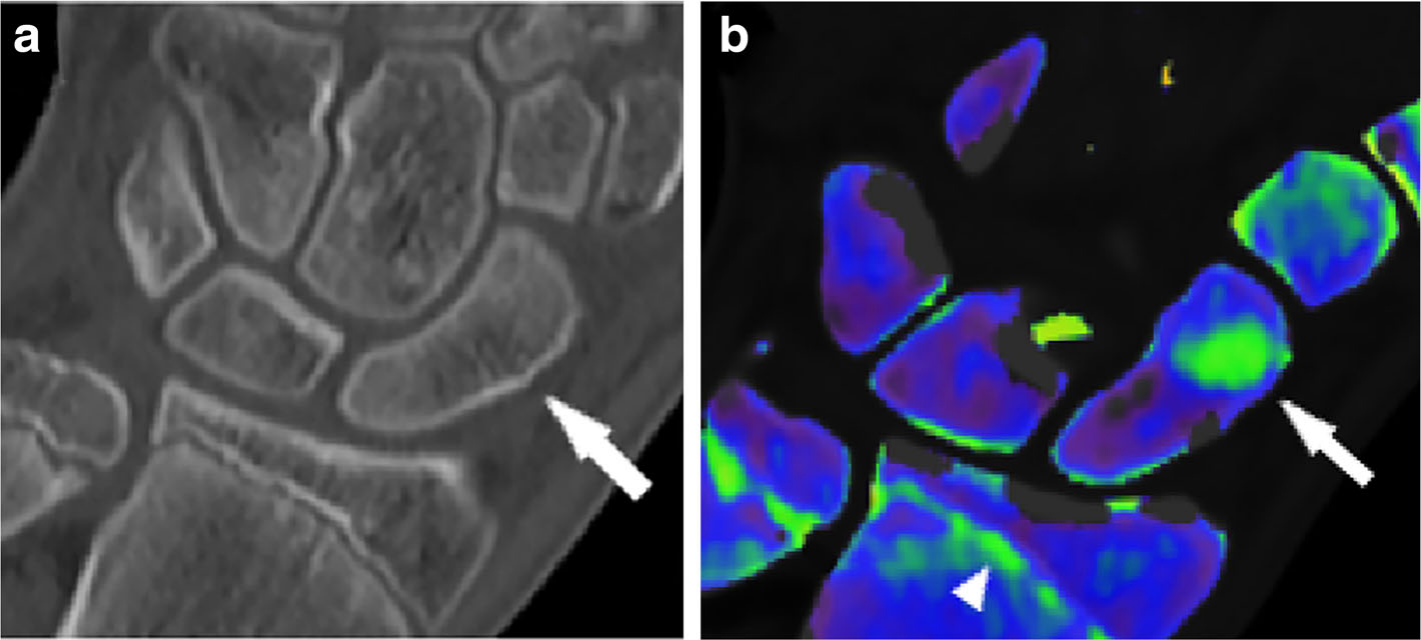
Hand dual-energy computed tomography scan in a patient with a non-displaced scaphoid fracture, which was confirmed at magnetic resonance imaging (not shown). Standard grayscale series (a) shows a subtle cortical interruption, which is not clearly suggestive for fracture (*arrow*). Color-coded virtual non-calcium image (b) depicts the presence of bone marrow edema confirming the hypothesis of a traumatic lesion. Of note, the epiphyseal line on the distal radius and ulna are also color-coded in green (*arrowhead*). From Dareez et al. [84]
